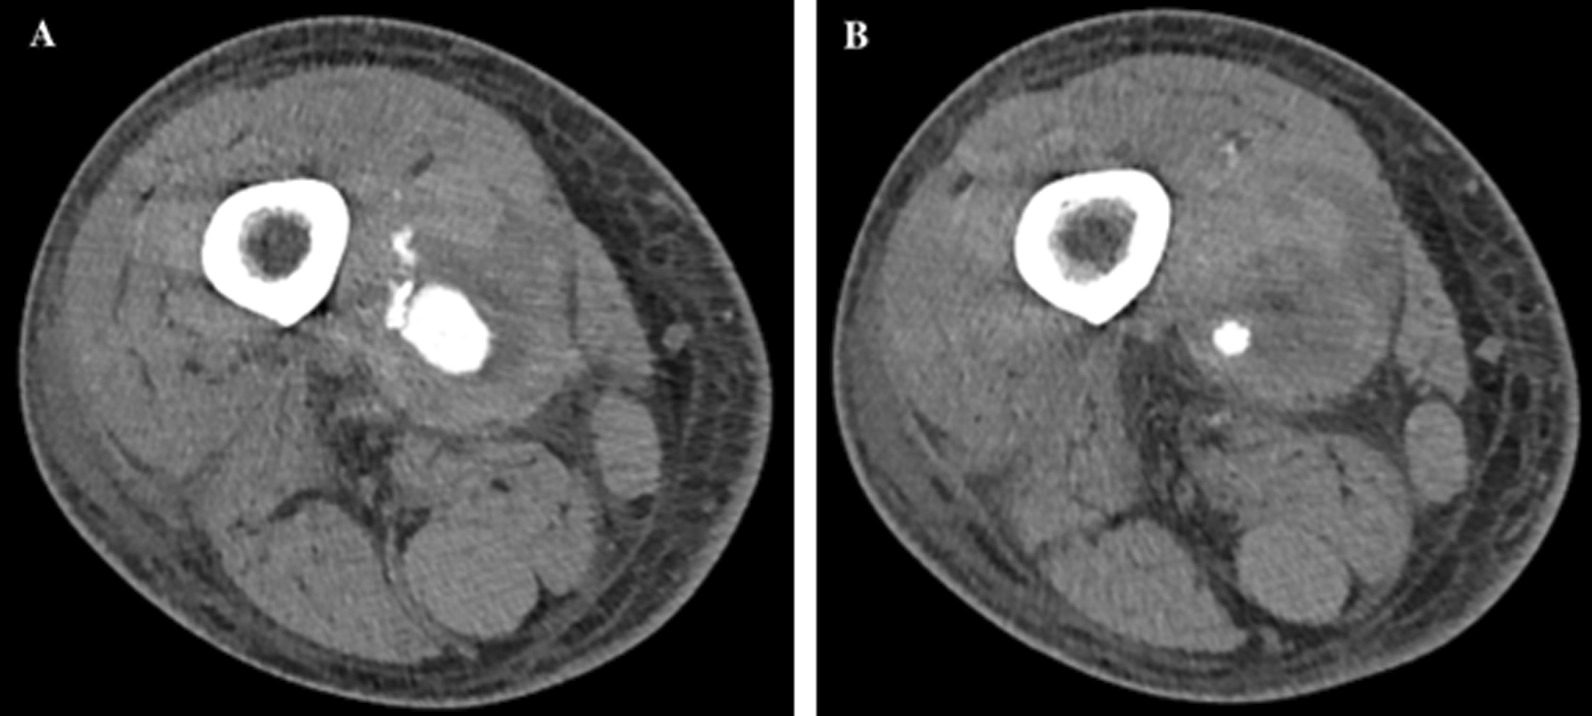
CT angiogram of right leg. CT angiogram with contrast of the right lower limb showing enhancement of the mycotic aneurysm (A) and hematoma formation (B)
